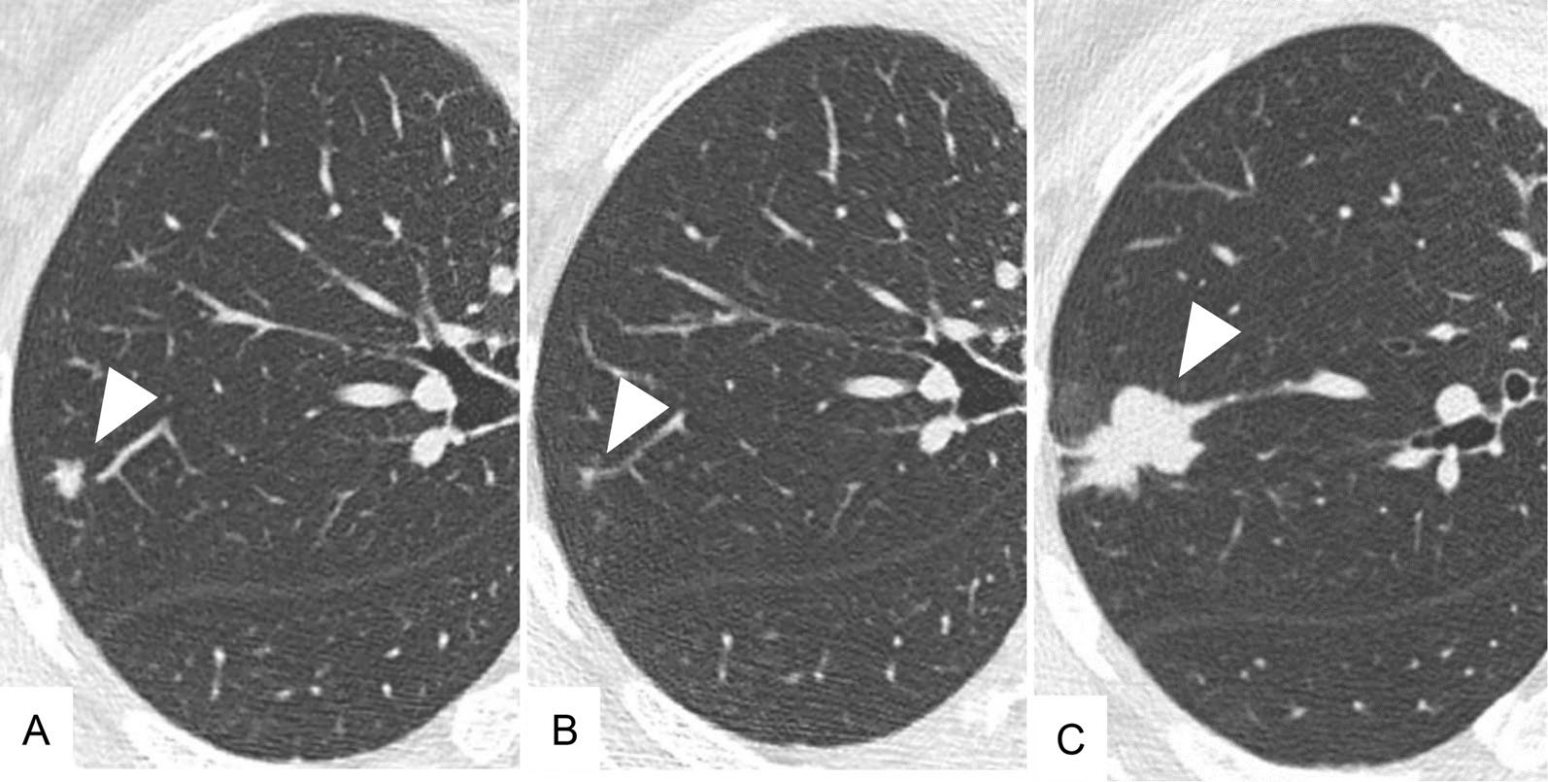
A A chest CT 7 years after radical surgery for the leiomyosarcoma showed a 4-mm irregular nodule (arrow). B The nodule regressed spontaneously to 2 mm after an additional 6 months. C The nodule regrew up to 24 mm over the next 5 years
